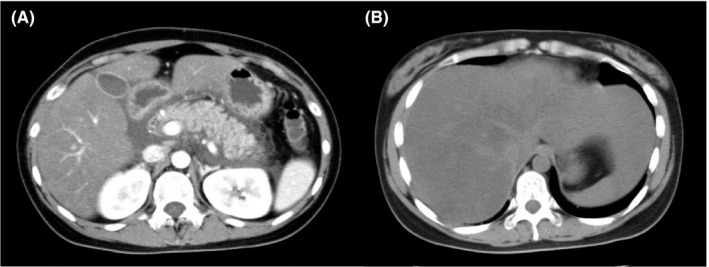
A, Enhanced abdominal CT revealed swelling of the pancreas with surrounding fat stranding and fluid accumulation. B, Plain abdominal CT revealed diffuse and large low‐density areas in the liver, suggestive of moderate to severe fatty liver
